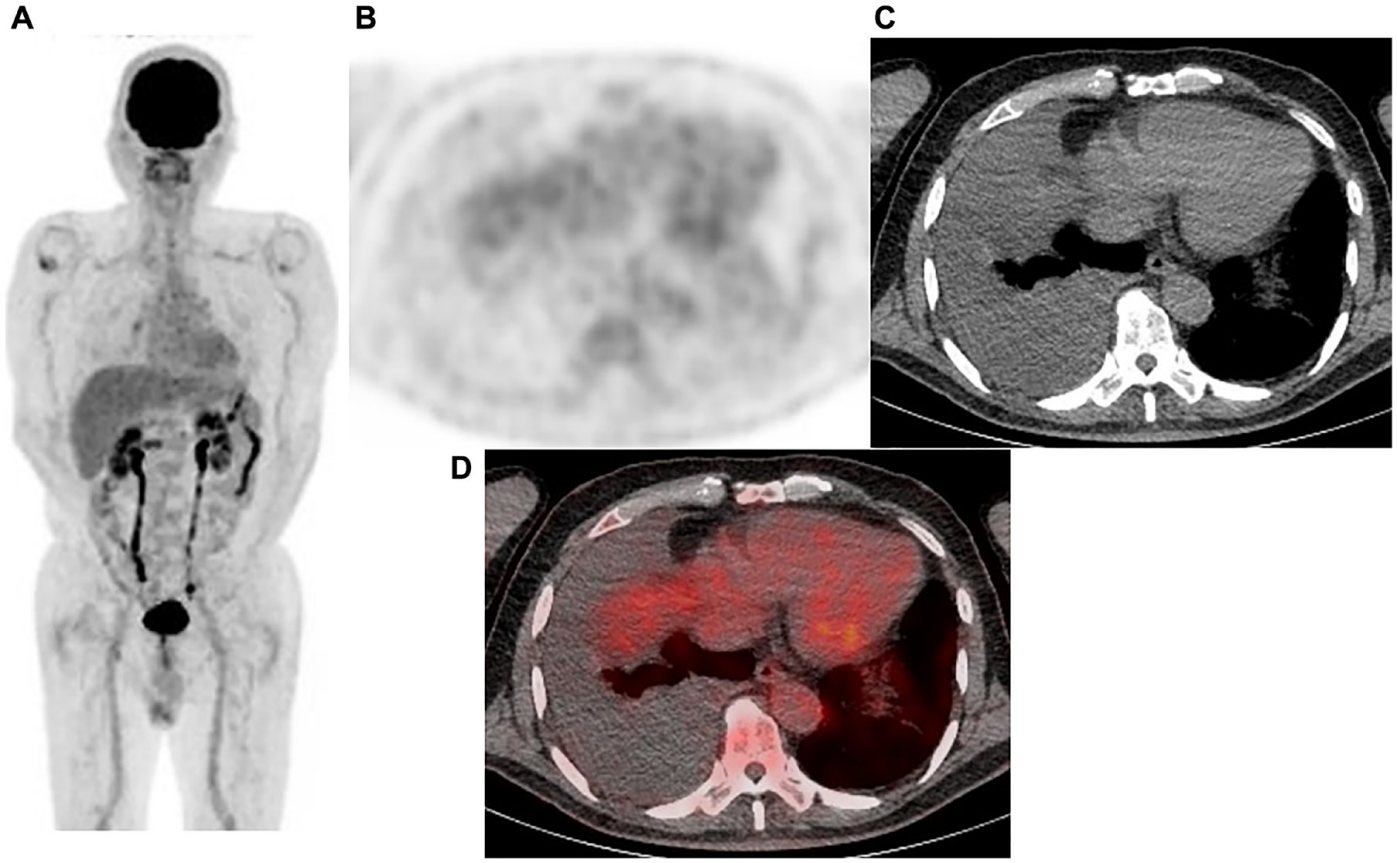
Representative case: 77-year-old male with right malignant pleural mesothelioma (epithelial type). (A) Maximum intensity projection (MIP) from FDG-PET, (B) Axial FDG-PET, (C) axial CT, and (D) fused FDG-PET/CT. Pleural effusion in the right pleural cavity was noted, while no FDG uptake was observed. The diagnosis was negative with protocol A (output value 0.127), B (grade 2), and C, whereas it was positive with protocol D (output value 0.448). VATS showed the sample to be positive, a malignant pleural mesothelioma (epithelial type).
